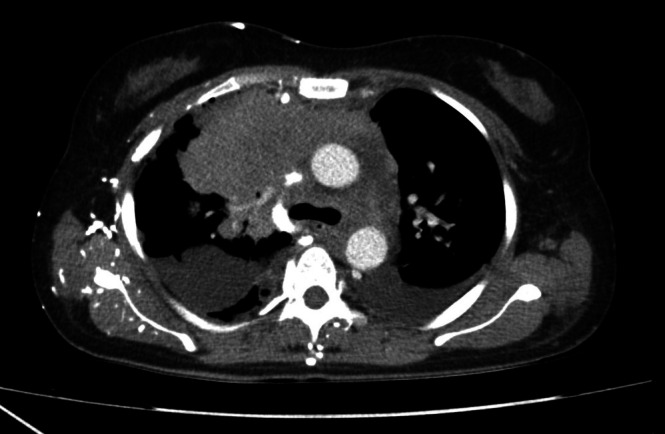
Chest CT scan revealing right upper lobe mass extending to the superoanterior mediastinum.
